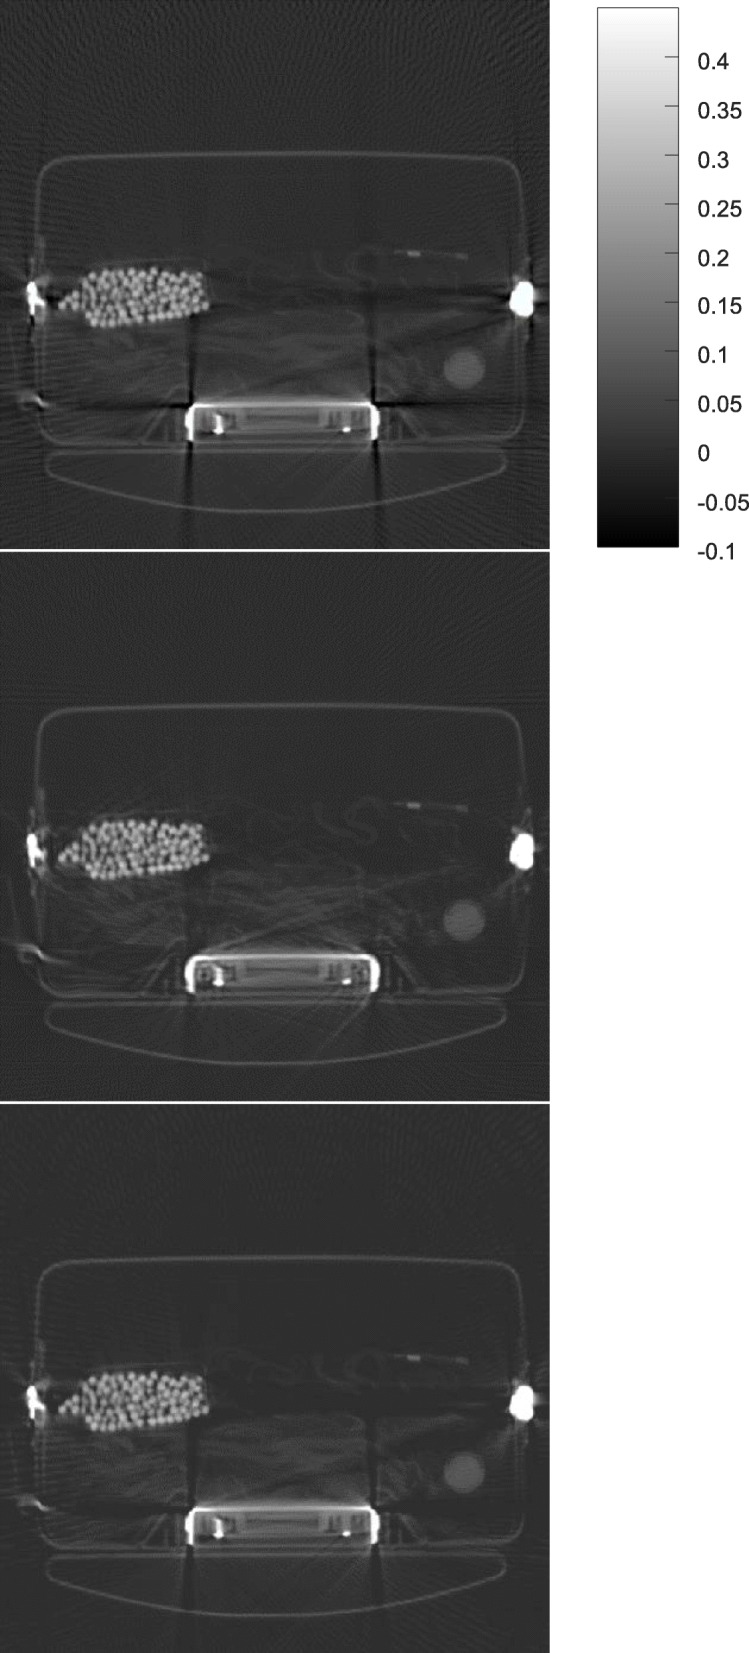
CT image reconstruction of airport bag #2. Top: the raw FBP reconstruction; Middle: the reconstruction using the proposed algorithm; Bottom: the raw FBP reconstruction with all negative pixel values replaced by zeros
